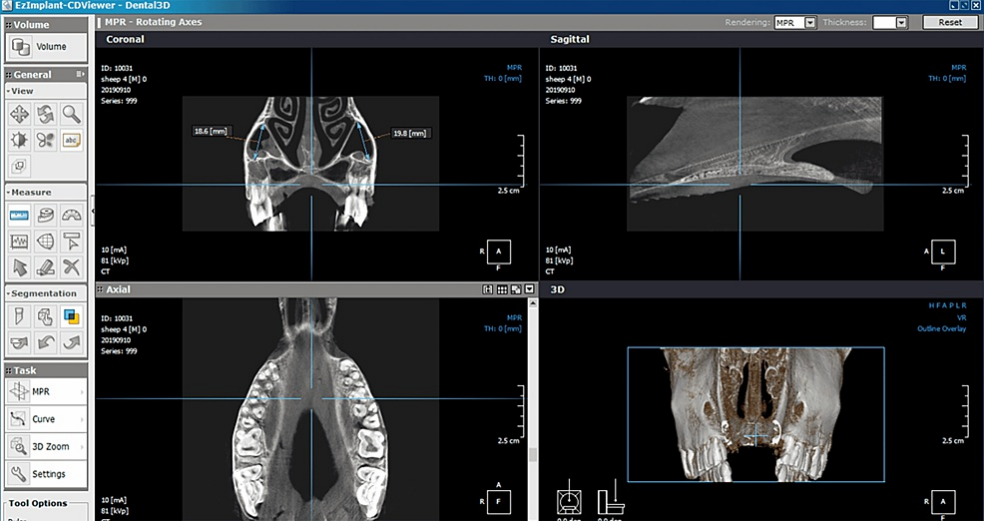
The measurement of the maxillary sinus height on the CBCT image CBCT: cone-beam computed tomography
